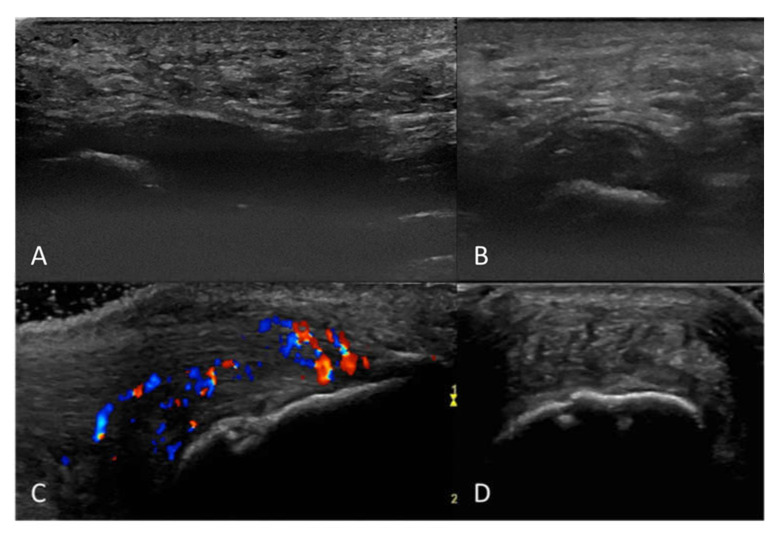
Greyscale images of the plantar fascia insertion with thickening and hypoechogenicity in longitudinal (A) and transverse (B) plane, and the Achilles tendon insertion with thickening, hypoechogenicity, color Doppler activity and enthesophyte in longitudinal plane (C) and greyscale image in transverse plane (D).
